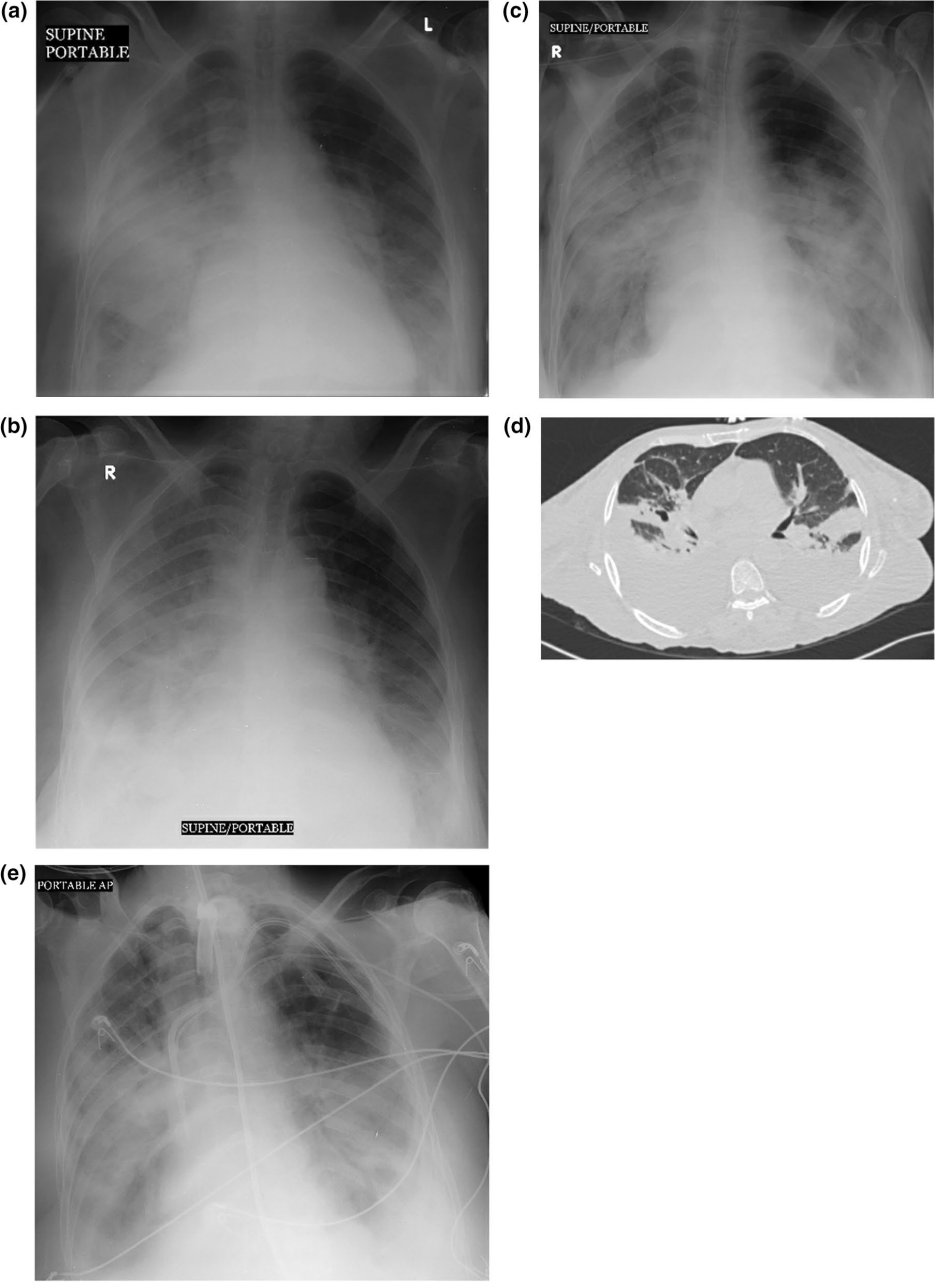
A 68-year-old man with Middle East respiratory syndrome coronavirus. Serial chest radiographs show a type 4 pattern of progression. A A frontal chest radiograph shows airspace disease involving the in the right upper, mid and lower zone with a chest radiographic score of 12 with bilateral pleural effusion. B On day 4, a follow-up frontal chest radiograph shows further deterioration of right lung air space opacity with increase of bilateral pleural effusion. C On day 6, further worsening of the bilateral air space opacities noted. D A CT scan done on the same day shows bilateral pleural effusion with bilateral air space consolidations. E On day 8, the patient further deteriorated and was put on ventilation. At this stage his serum LDH was 1326 and he died on day 10
